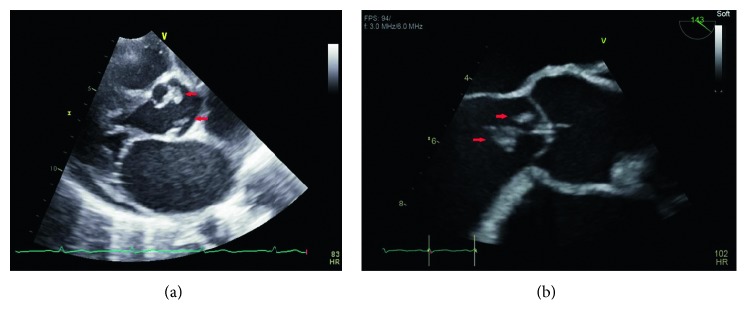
Large aortic valve vegetations on echocardiogram, shown in red arrows.
